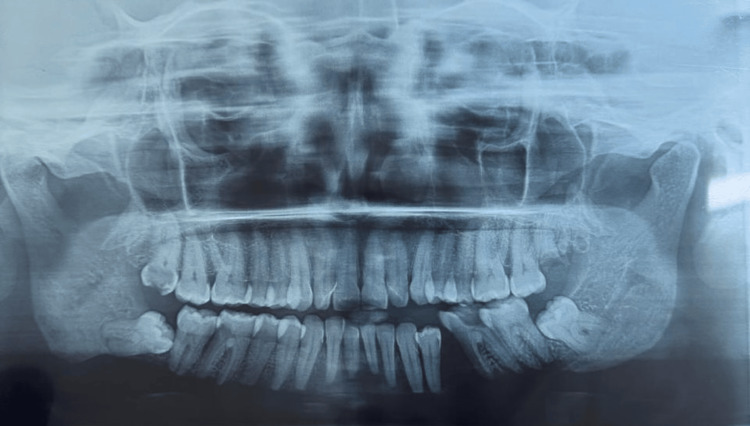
Panoramic radiograph image showing well-defined radiolucency extending from the right angle of the mandible below the roots of the right third molar to the body of the mandible below the left second molar, suggesting complete bilateral resorption of the lower border and mental process of the mandible. No resorption of roots is visible
